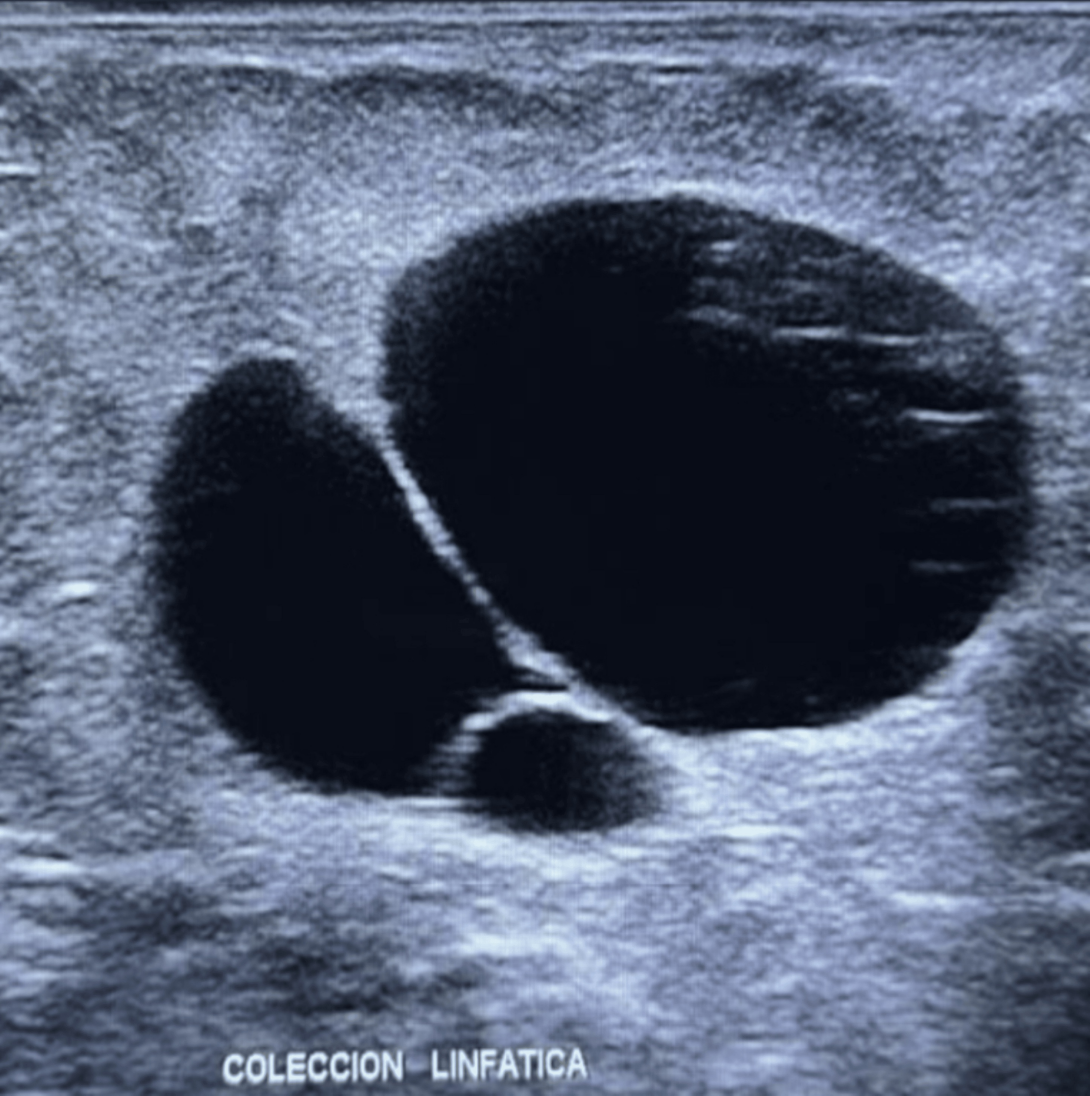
Ultrasound showing remnant cystic collection in the right groin three years after surgery.
